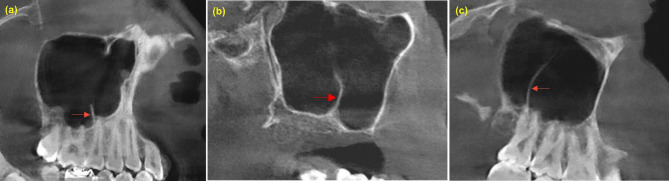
Sagittal CBCT sections showing several septa locations (a) anterior, (b) middle, (c) posterior
